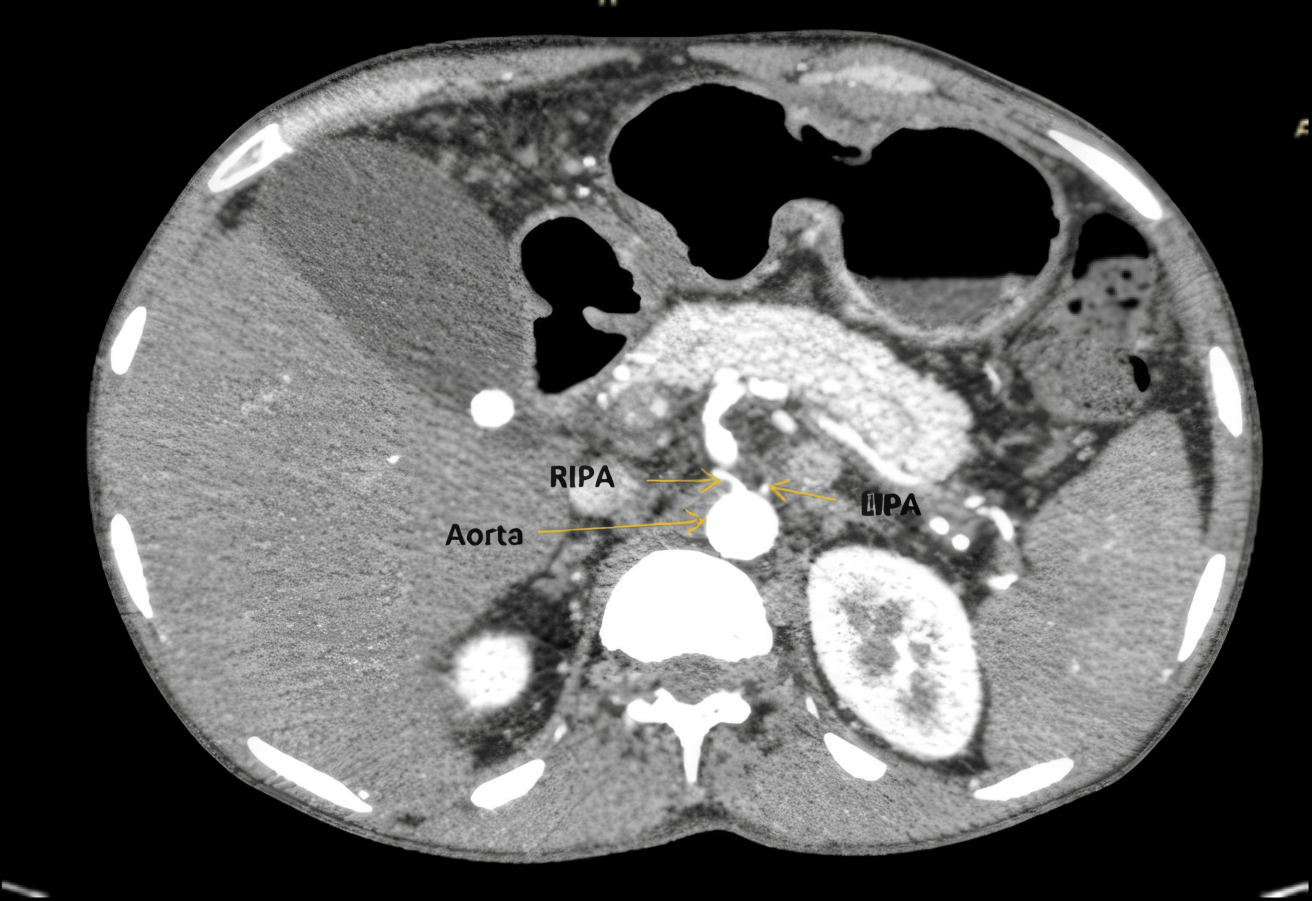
Axial CT image showing bilateral normal origin of aortic IPAs IPA, inferior phrenic artery; LIPA, left inferior phrenic artery; RIPA, right inferior phrenic artery
